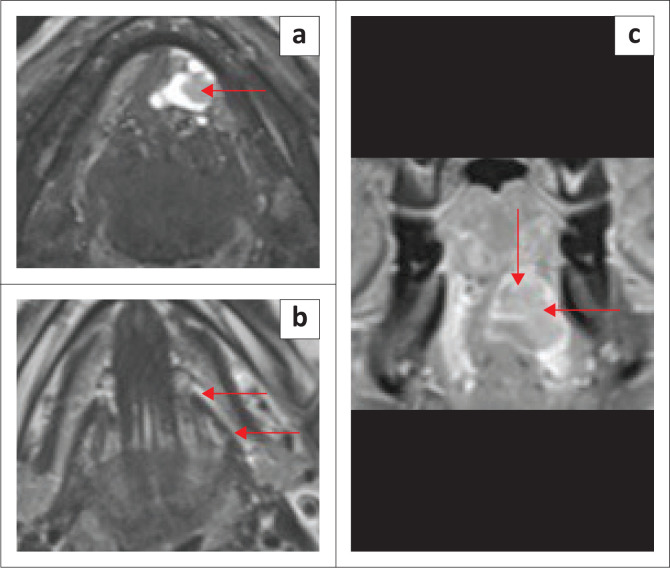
Mucoepidermoid carcinoma of the salivary gland in the left anterior floor of mouth/sublingual space, in an adult patient. A tumour is obstructing the opening of left submandibular duct. T2-weighted short tau inversion recovery (T2W-STIR) axial image (a) shows the slightly hyperintense tumour projecting into left submandibular gland duct opening (red arrow). The T2 axial (b, red arrows) shows the dilated left submandibular gland duct. The T1 contrast-enhanced fat-suppressed coronal image (c) demonstrates the enhancing tumour (red arrows).
